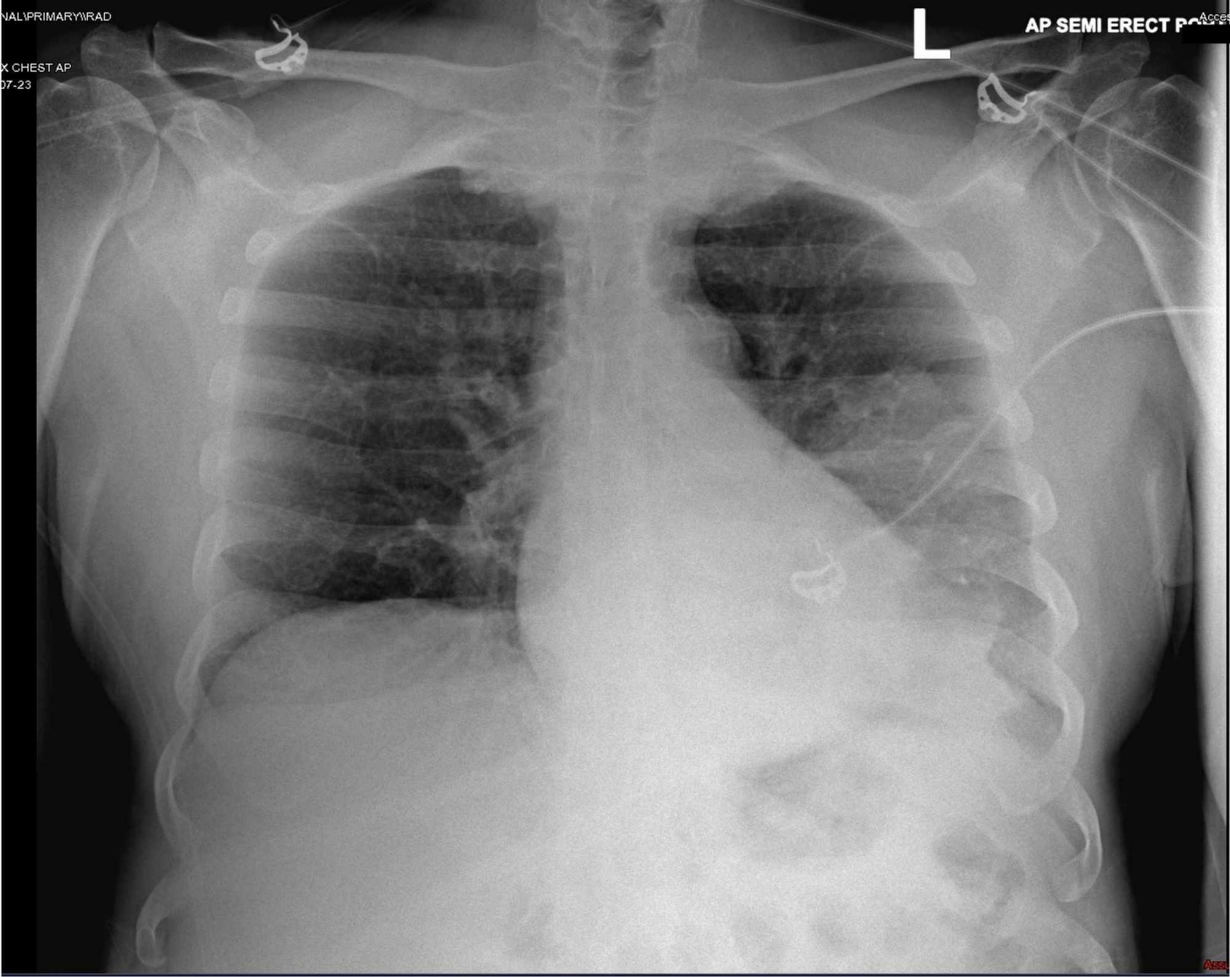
Chest X-ray demonstrating left lung opacity
